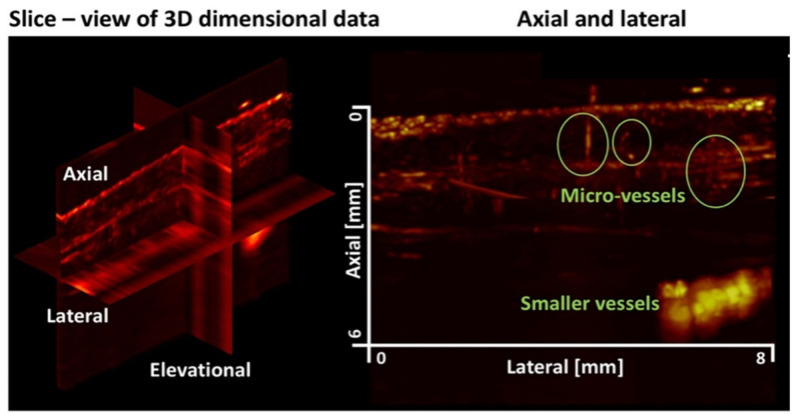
Using HFUS, the image shows volumetric visualization of dermal vasculatures on the dorsum of the hand with a new region-based SVD clutter filtering. Rendered in the slice-view and volumetric view to locate the vasculature along the volume in maximum intensity projection images. This image was published under open access, CC-BY 4.0 rights, and originates from Volumetric Visualization of the Dermal Vasculature with Signal and Image-based Feature Extraction on a High-frequency Ultrafast Ultrasound Dataset; [37] Seven studies reported the use of advanced Doppler-based techniques (SMI, MV-Flow, MicroV, and AP), describing enhanced sensitivity to low-flow velocities compared with conventional Doppler ultrasound, while noting limitations in visual representation relative to some other modalities (Figure 4) [9,10,11,35,36,40,41].
